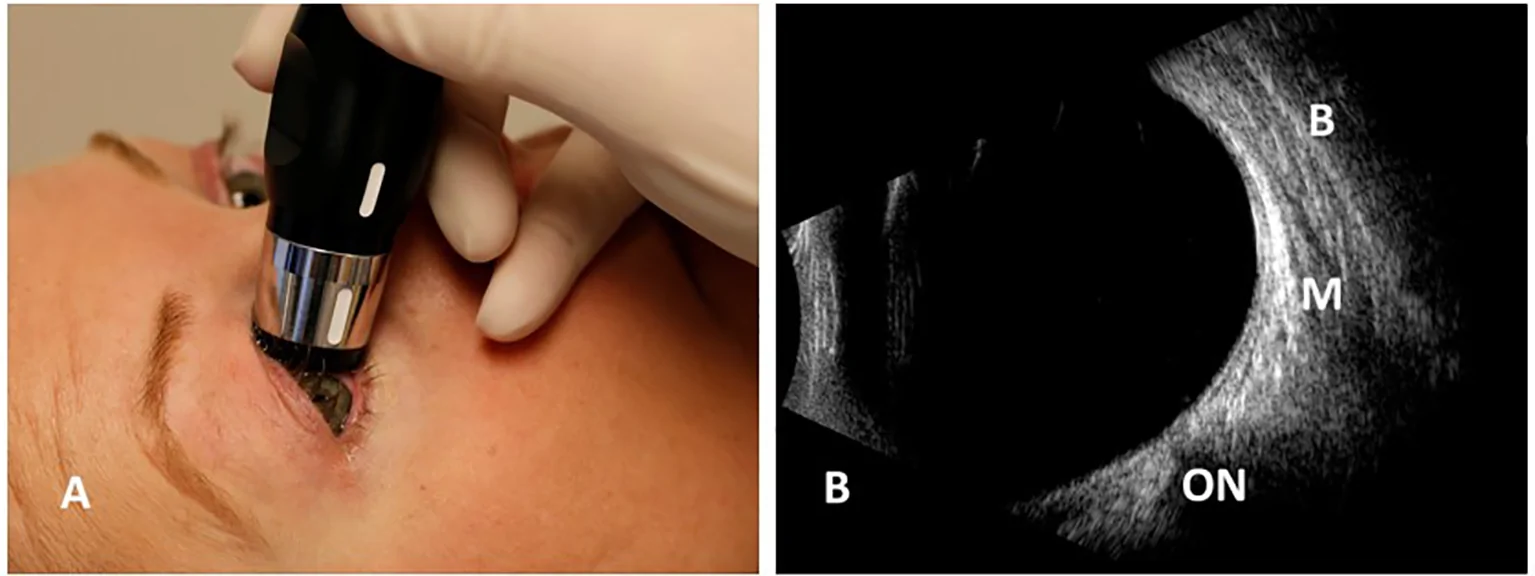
Longitudinal scan aimed temporally. (A) The patient is in right gaze, and the probe is placed on the nasal limbus with the marker oriented towards the cornea with the sound sweeping along a specific meridian of the temporal fundus. (B) The corresponding sonography of a normal eye and orbit in longitudinal view, where the optic nerve and posterior pole are imaged on the lower portion of the echogram, while the fundus periphery is imaged on the upper portion of the ultrasound. *M*, muscle; *ON*, optic nerve; *B*, lateral bone wall.
